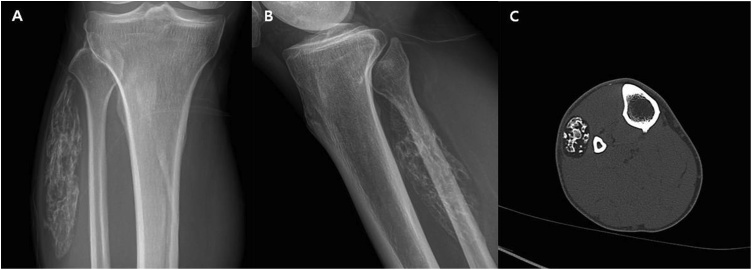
Plain X-ray (A,B) and CT (C) scan showing an ossified soft tissue mass. The density of the mass was similar to the subcutaneous fat. Diffuse ossification is seen in the center. Continuity between a tumor and fibula was not found.
